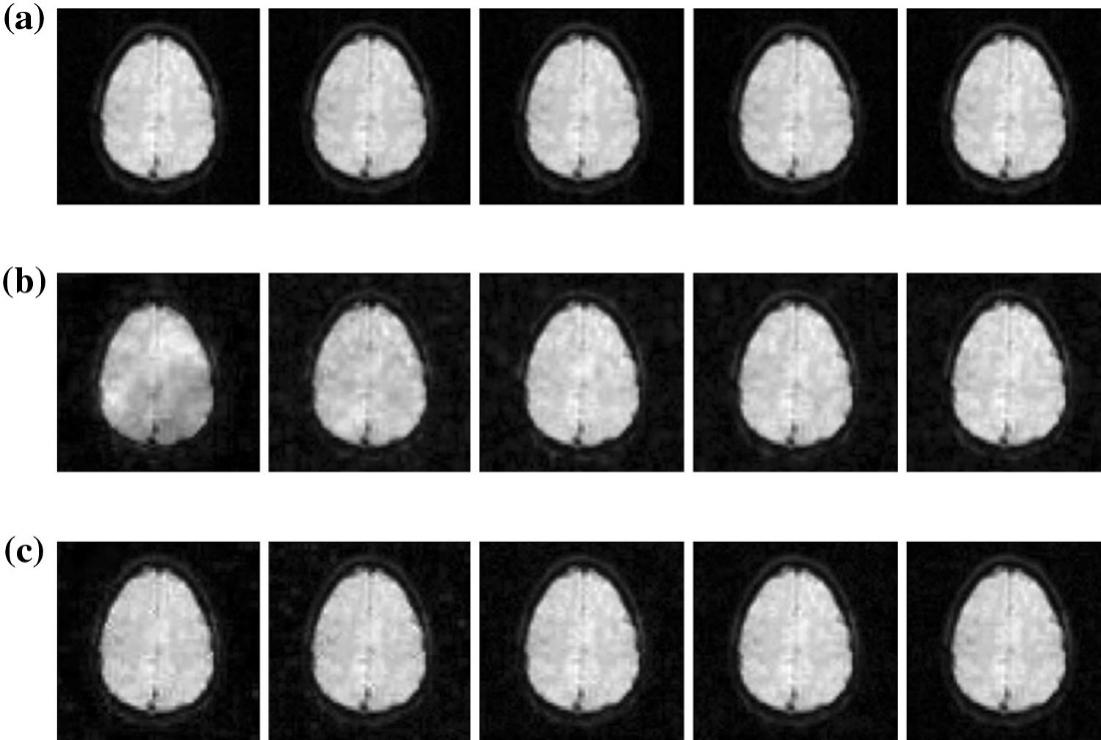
Reconstructions of functional MR images (2nd,  5th,  8th,  11th,  14th frames). a original sequence, b random sampling, c proposed method
